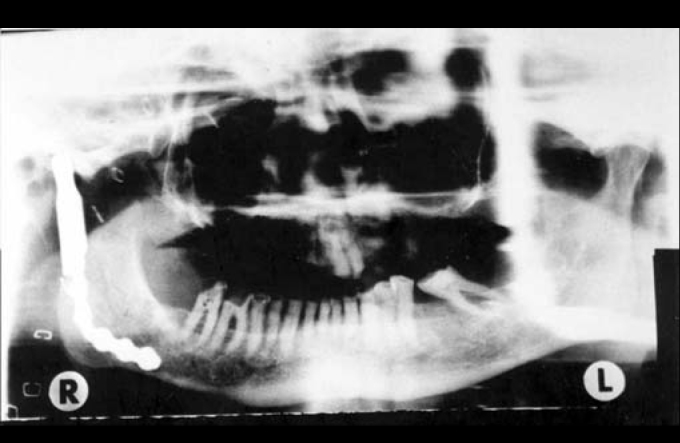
Panoramic X-ray, ten months postoperatively
